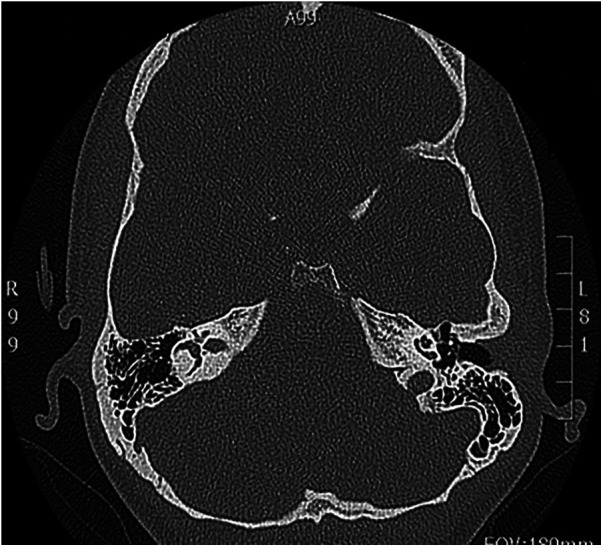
CT scan of temporal bones. Regular pneumatisation, regular configuration of ossicular chain.
